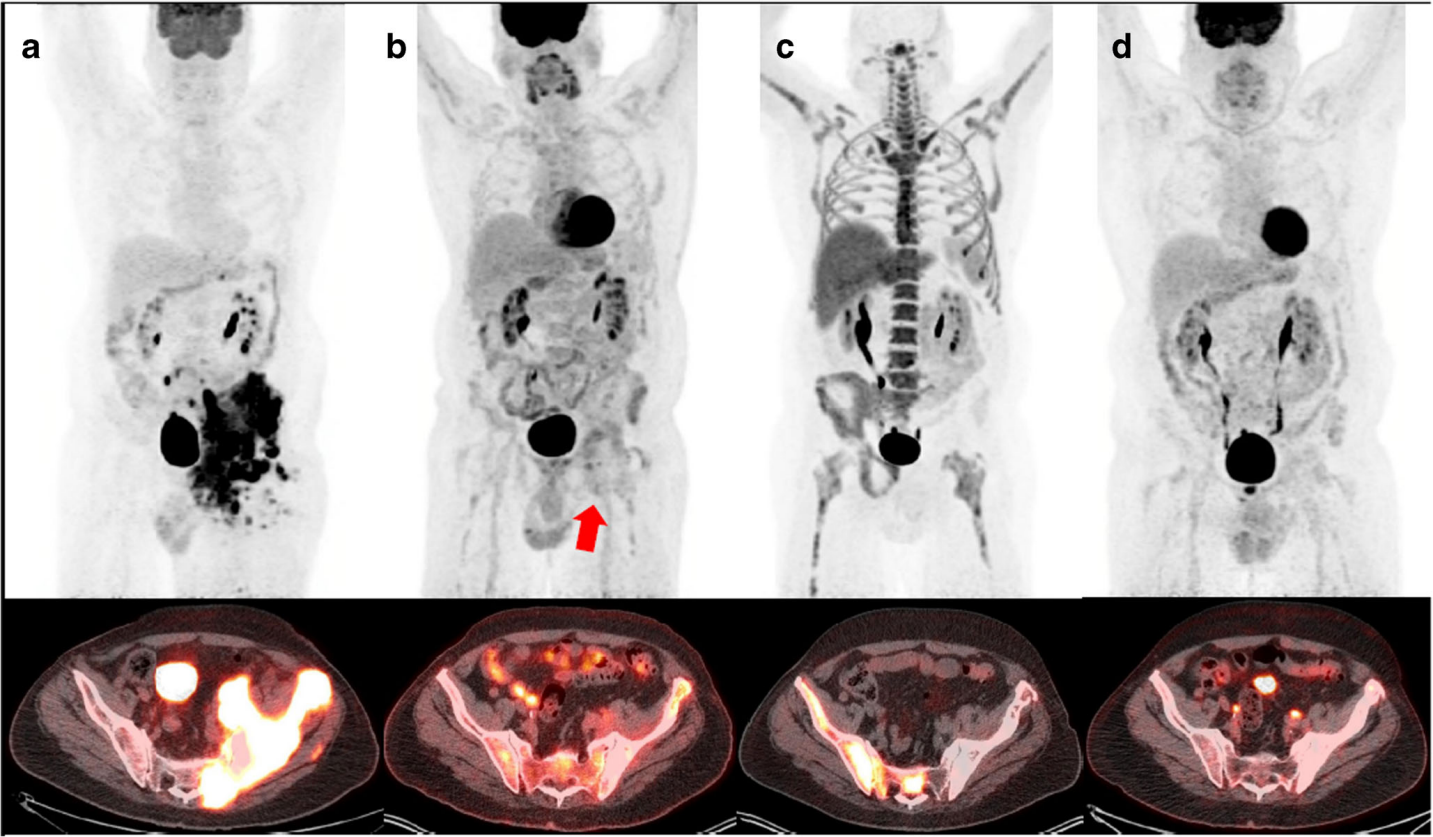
Images in 46-year-old man. a Baseline FDG PET maximum intensity projection (upper row) and axial PET/CT fusion image (lower row) show intense FDG uptake in a bulky mass within the left pelvis. b Interim FDG PET maximum intensity projection (upper row) and axial PET/CT fusion image (lower row) show decreased activity, but the lesion remained positive (arrow) due to an area with visually higher than liver FDG uptake. c Interim FLT PET image (upper row) and axial PET/CT fusion image (lower row) show photopenic tracer uptake in this lesion, which was considered negative for disease. d End-of-treatment FDG PET maximum intensity projection shows (upper row) and axial PET/CT fusion image (lower row) substantial reduction in FDG activity which is visually same with mediastinal FDG uptake. This patient remains without evidence of recurrence for 3 years from initiation of treatment
